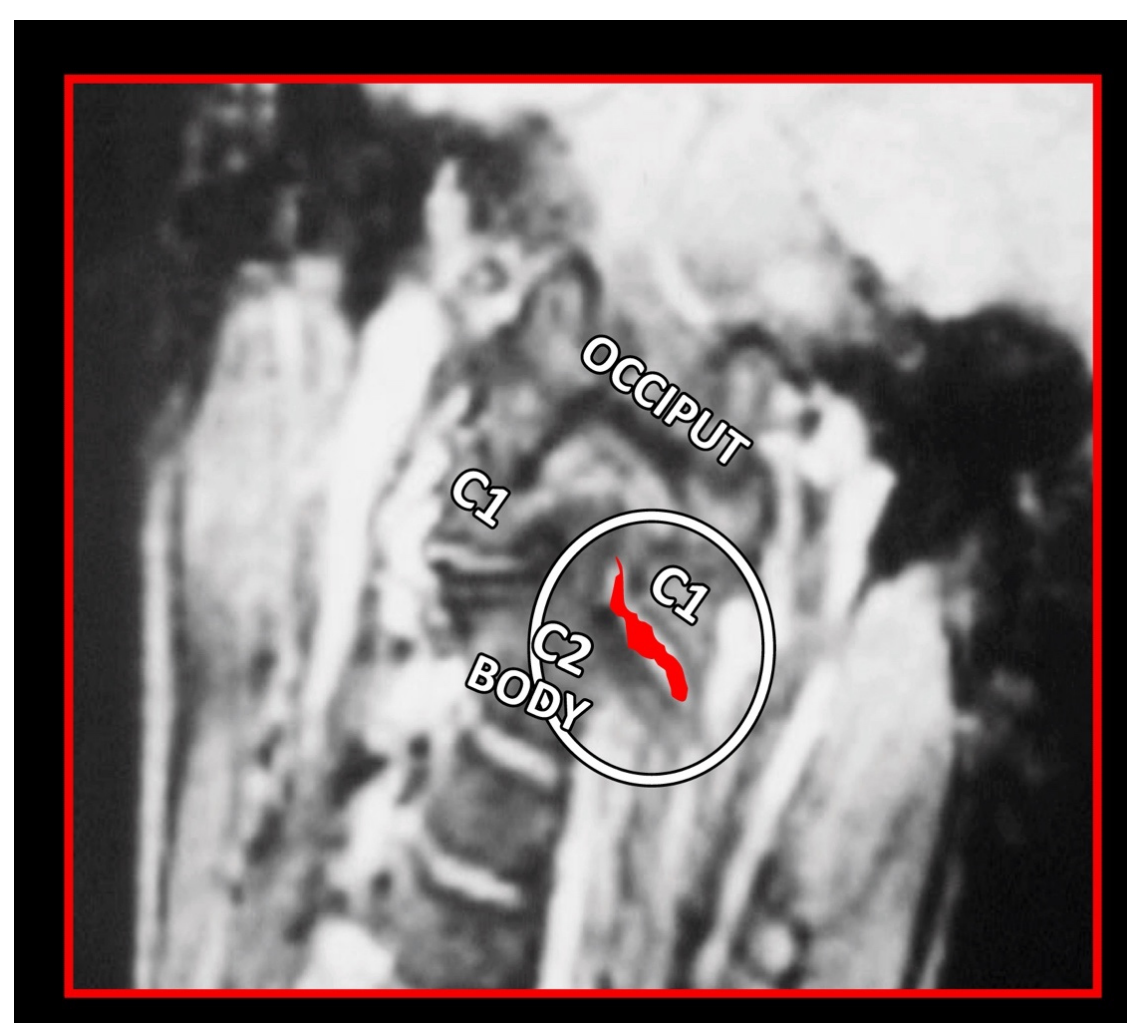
Dynamic coronal magnetic resonance imaging study. Lateral instability of *atlas*-*axis* segments. The study facilitates the understanding of the dysfunction to plan the treatment process and restore left rotation of the *axis* on the C3. Maintain proportional rotational patterns of movement between the *atlas* and *axis*.
